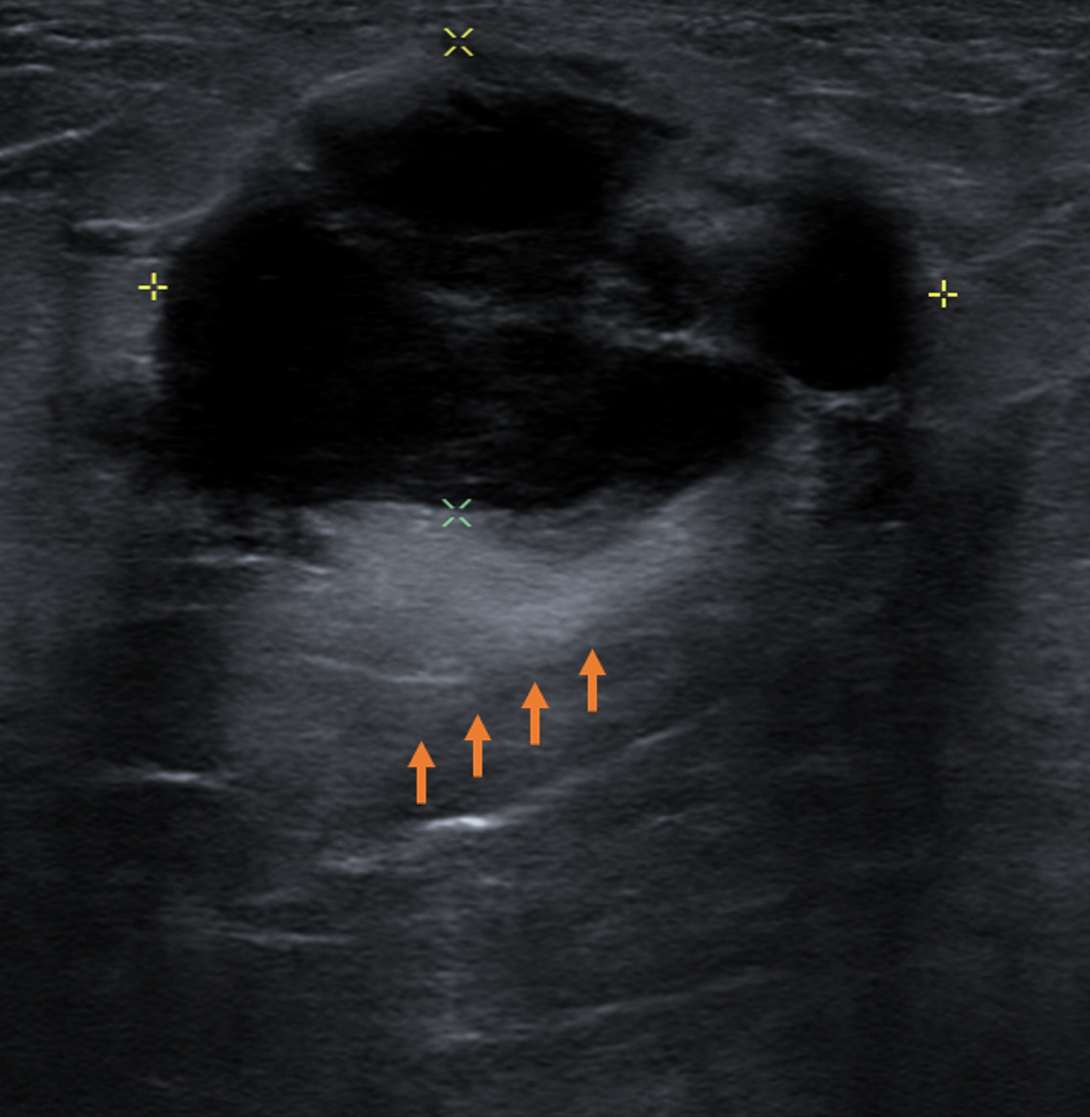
Targeted ultrasound of the left breast Targeted ultrasound of the left breasts demonstrates a complex solid and cystic mass at the 3:00 position, 5 cm from the nipple. There is a posterior acoustic enhancement (orange arrows). This should not be confused with a "complicated cyst." Complex solid and cystic masses should be biopsied (BI-RADS 4). BI-RADS, Breast Imaging Reporting and Database System score.
